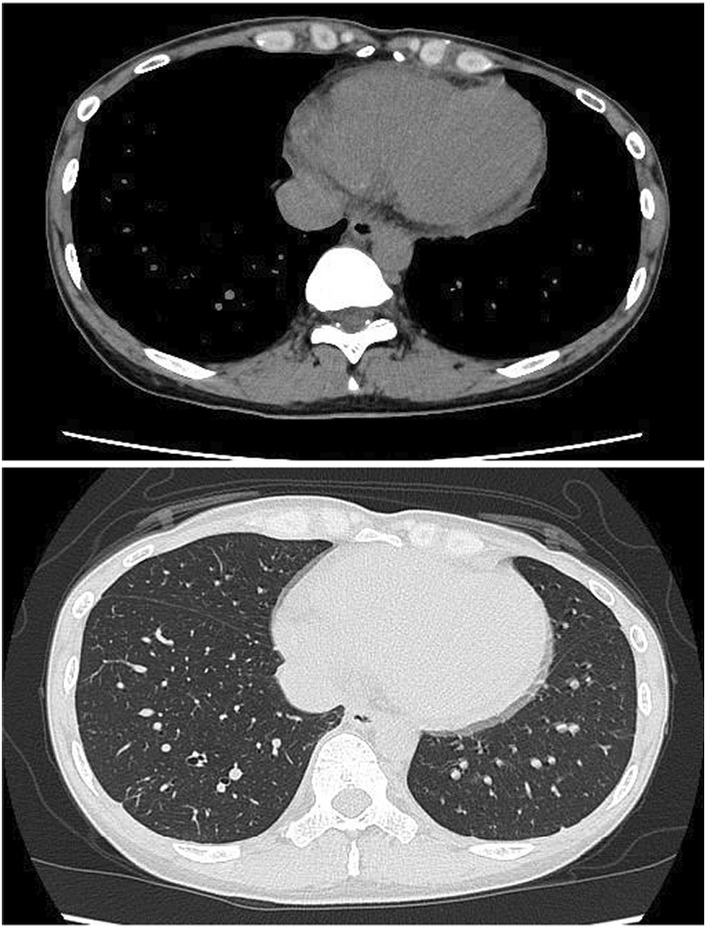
Chest computed tomography after treatment showing decreased pericardial effusion.
